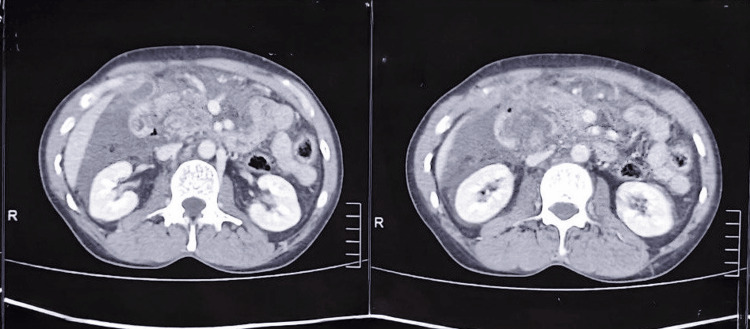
Grade 5 pancreatic injury in contrast CT abdomen
